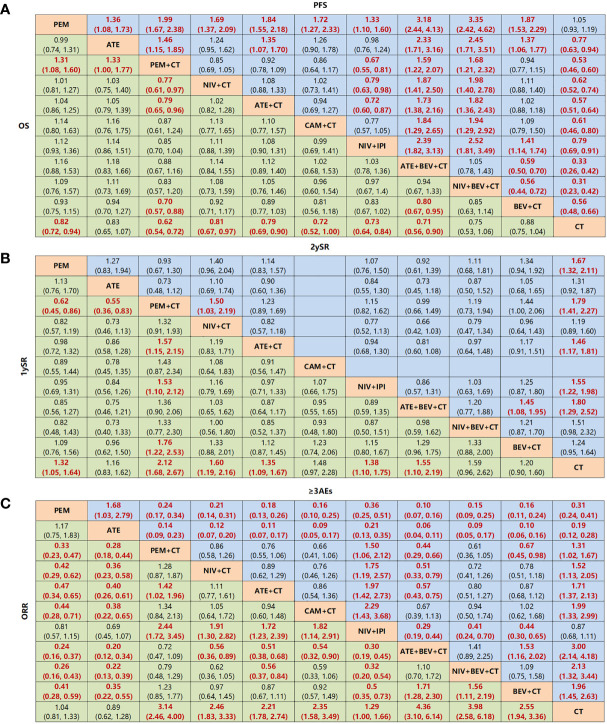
Network meta-analysis of specific immuno-related regimens with long-term survival in overall population. (A) Pooled HR (95% CrIs) for overall survival (OS) and progression-free survival (PFS) in comparisons of all treatment strategies. (B) Pooled OR (95% CrIs) for 1-year survival rate and 2-year survival rate in comparisons of all treatment strategies. Data in each cell are HR or (95% CrIs) for the comparison of row-defining treatment versus column-defining treatment. HR less than 1 and OR more than 1 favor upper-row treatment. Significant results are highlighted in red and bold. (C) Pooled OR (95% CrIs) for ORR and ≥3 AEs in comparisons of all treatment strategies. PEM, pembrolizumab; ATE, atezolizumab; NIV, nivolumab; CAM, camrelizumab; CEM, cemiplimab; BEV, bevacizumab; IPI, ipilimumab, CT, chemotherapy.
